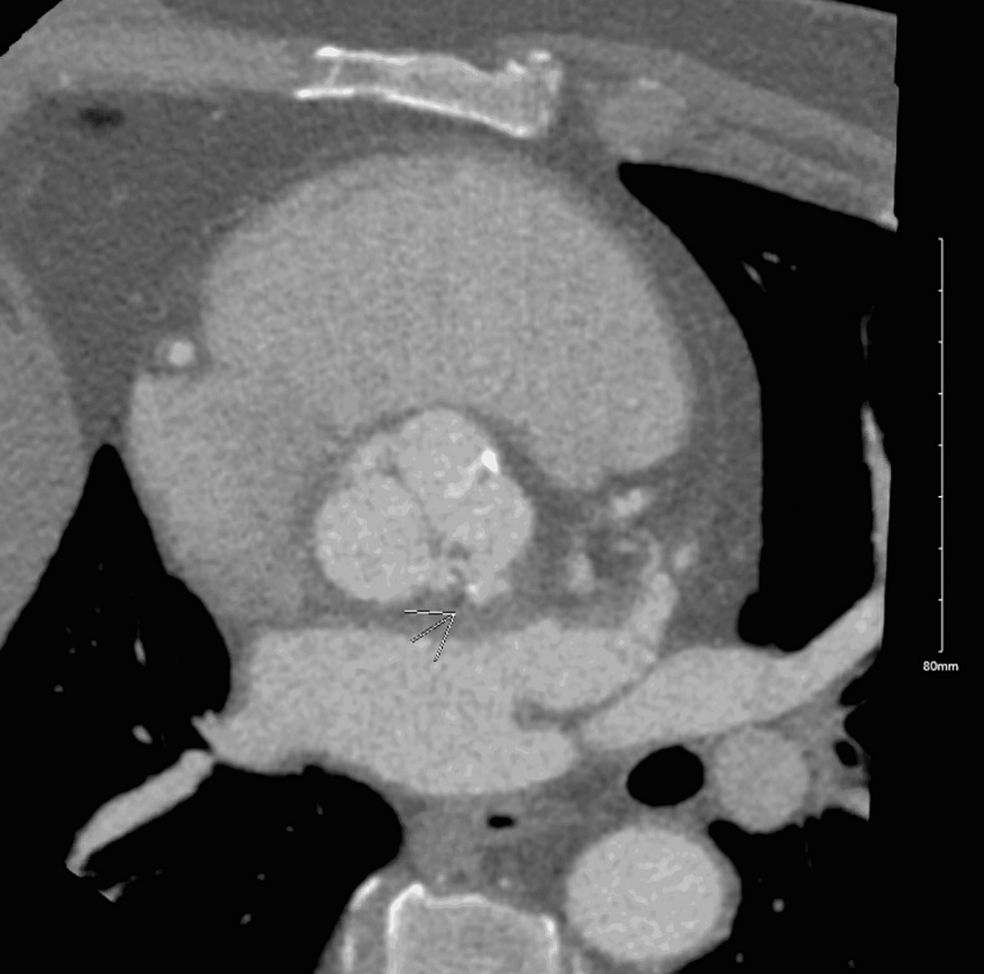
Cardiac axial section CT at the level of the aortic valve with an arrow showing peri-valvular abscess
